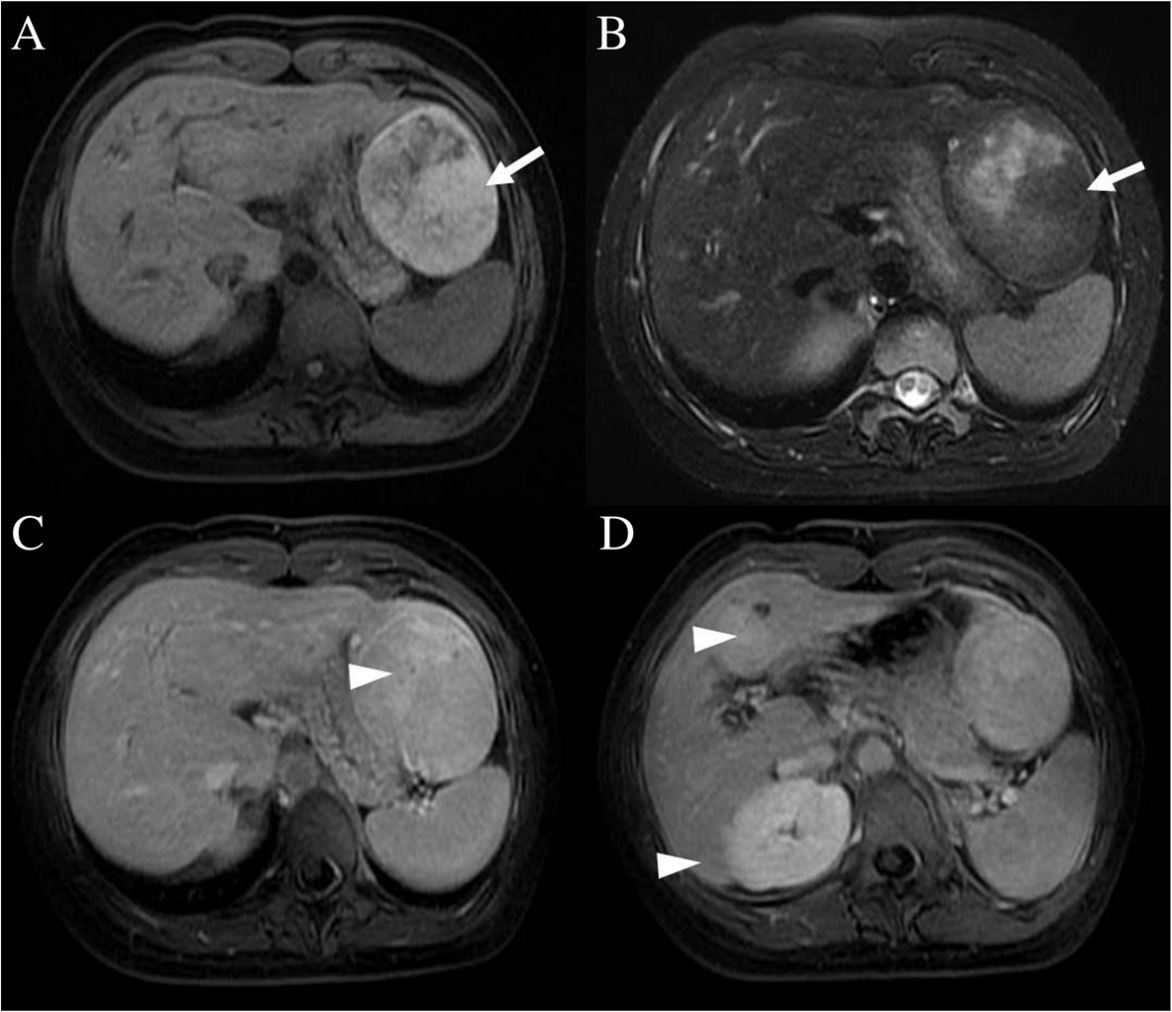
Abdominal precontrast magnetic resonance imaging (MRI) show lesions in segment II. Most of the tumor in segment II has heterogeneously high signal intensity on both fat suppressed T_2_-weighted images (A, white arrow) and T_1_-weighted images (B, white arrow). On delayed-phase images after gadopentetate dimeglumine administration, central delayed enhancement was noted in all three tumors (C and D, white arrowheads).
